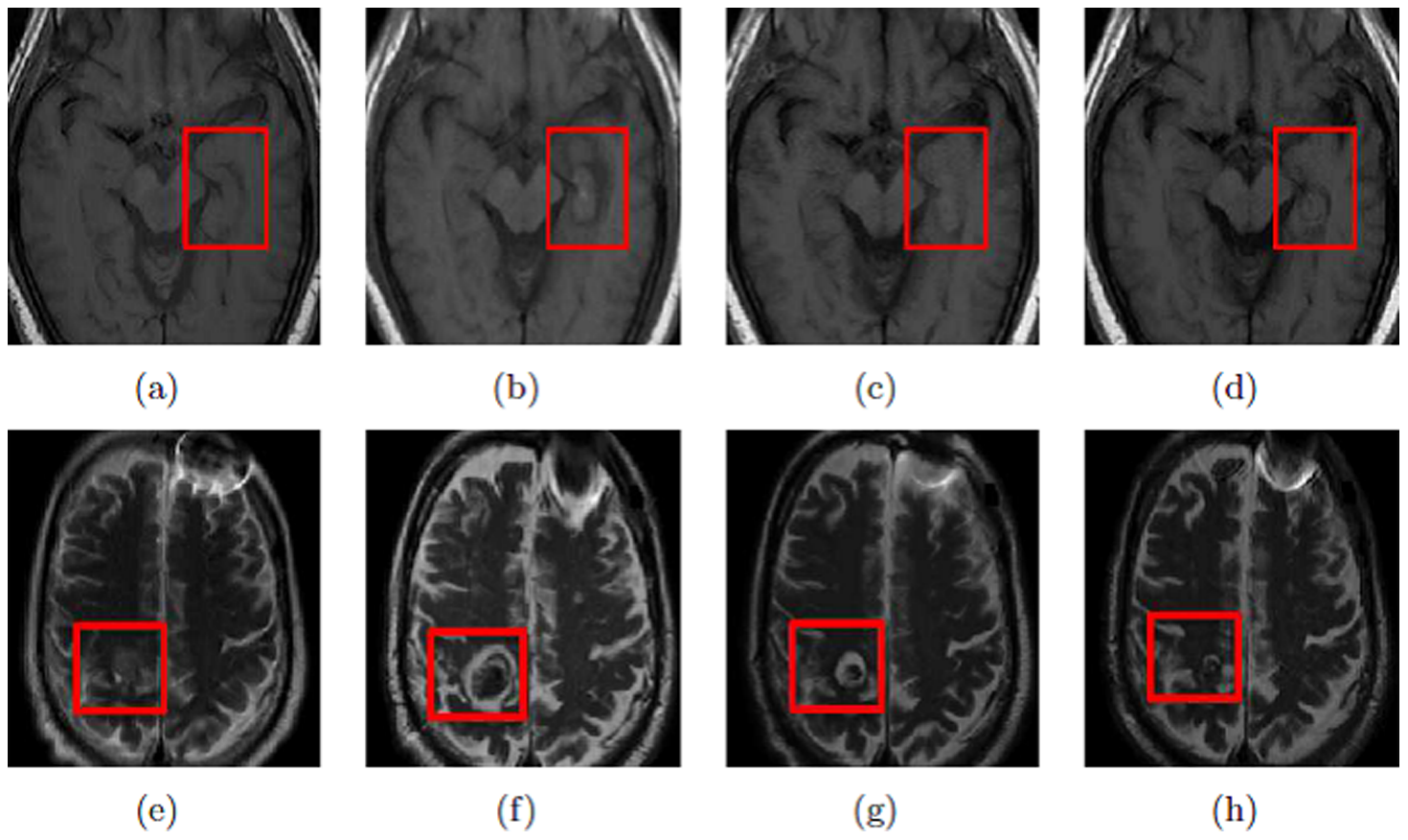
(a)–(d) show a 2D T1w MRI slice at different time points, pre-LITT (a), 24- hours post-LITT (b), 1-month post-LITT (c), and 3-month post-LITT (d) for a seizure-free epilepsy study, while 1(e)–(h) show a 2D T2w MRI slice at pre-LITT (e), 24-hours (f), 2-months (g), and 7 months (h) respectively for a successfully treated (no signs of recurrence at the time of evaluation) GBM study. Note how changes in imaging markers subside over time in the case of a LITT procedure with successful treatment.
